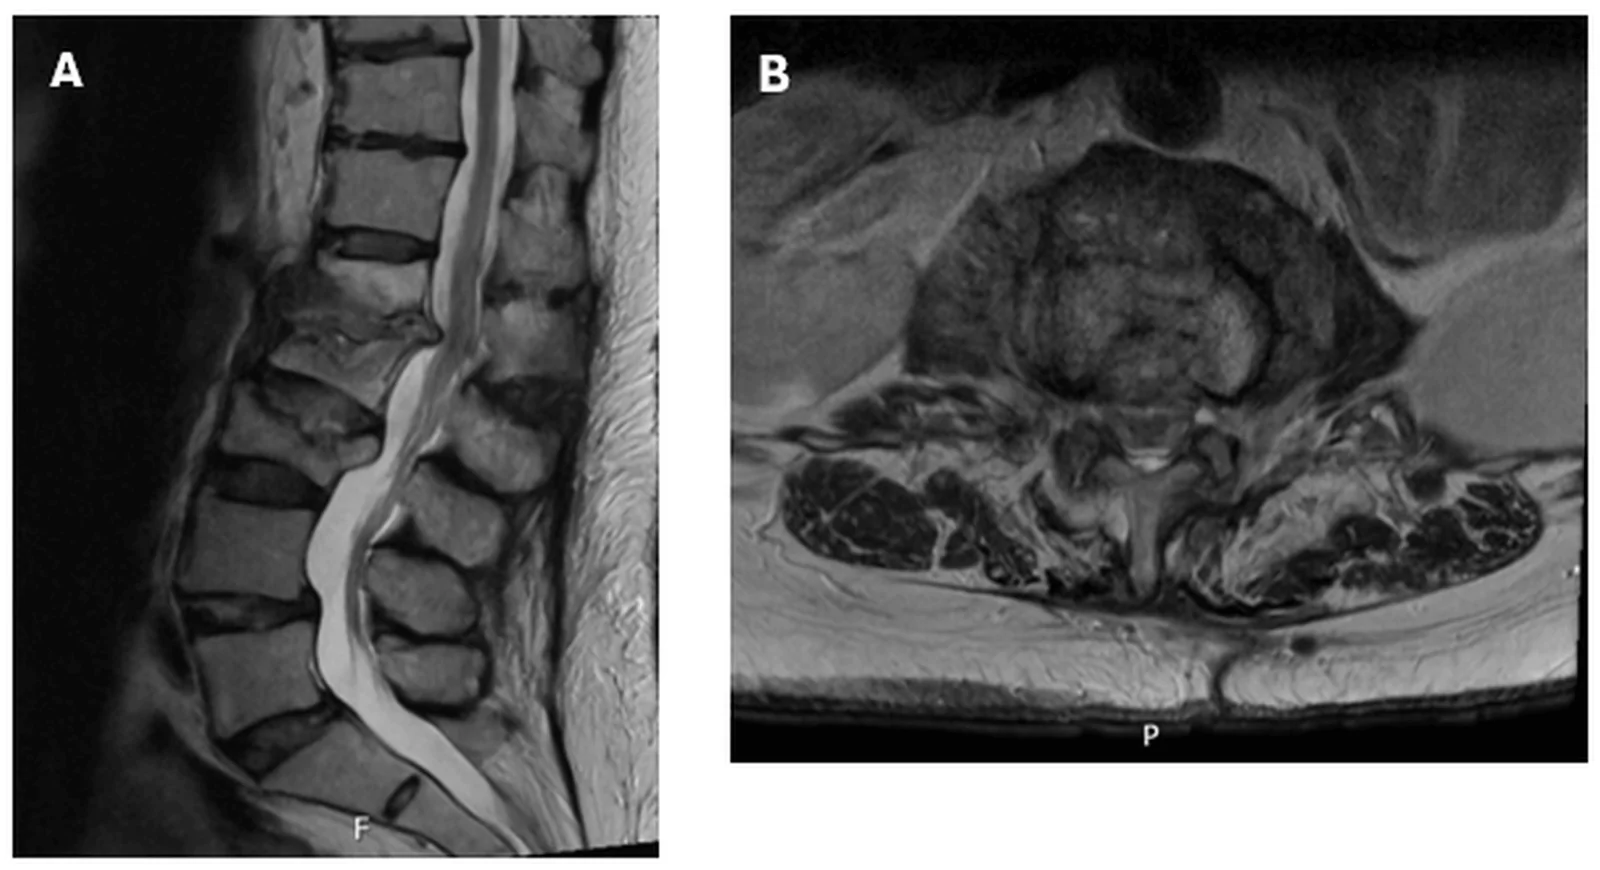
(A) Preoperative sagittal MRI demonstrating progressive height loss of L1 and L2 with inflammatory and destructive changes at L1-L2 consistent with persistent osteomyelitis and discitis; abnormal signal at the L3 superior endplate, of uncertain significance (granulation tissue versus infection), is also visible; and (B) Axial MRI demonstrating the inflammatory and destructive changes at L1-L2 consistent with persistent osteomyelitis and discitis.
